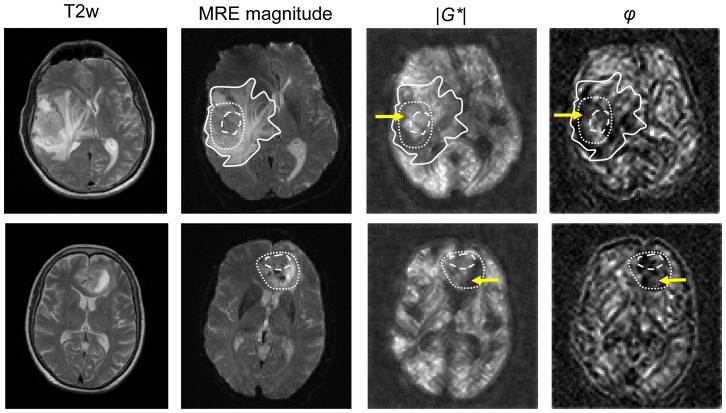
Anatomical T2-weighted images (T2w), MRE magnitude images, and 3DMMRE parameter maps (*|G*|* and *φ*) of 2 GB patients (upper row: patient #15, bottom row: patient #14, corresponding to the tables). The selected regions of tumor (dotted lines) and edema (solid line in #15) were used for the parameter evaluation as given in Table 2. The region of HAM is indicated by the dashed line. The yellow arrows indicate compartments of soft tissue properties (low *|G*|*) but different dissipative behavior (*φ*) in both tumors. |G*| was scaled from 0 to 3 kPa, φ was scaled from 0 to 2.5 rad.
